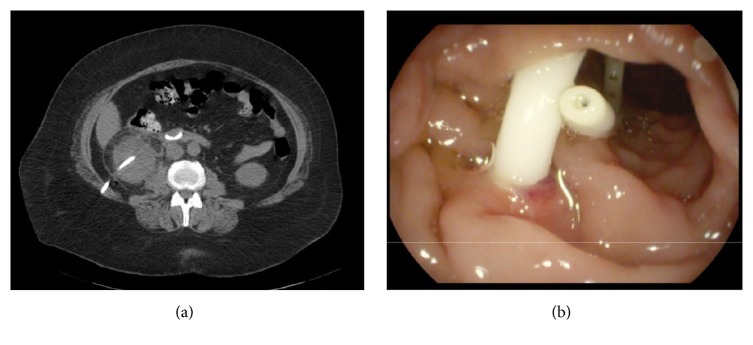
(a) CT. Percutaneous drain in the second part of the duodenum. (b). Pigtail of the percutaneous drain in the second part of the duodenum.
